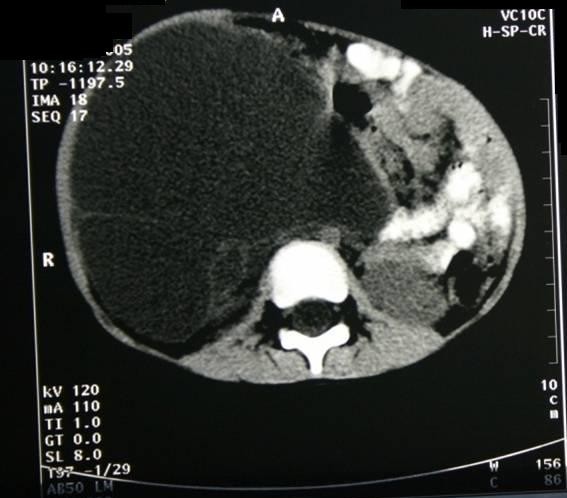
CT scan shows a well-defined retroperitoneal thin-walled, multilocular cystic mass with septa and fluid attenuation.
